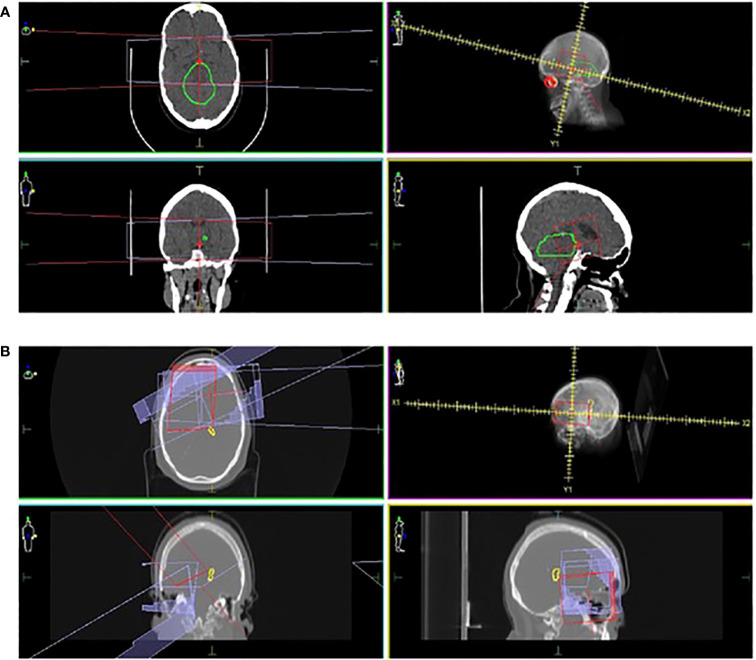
Locations of second brain malignancies relative to the treatment field. CT images in three planes, radiation treatment plans and the locations of second brain tumors are shown. In one patient (A), the second tumor (marked as green) is observed within the radiation field (marked in red and purple). In a second case (B), an anaplastic astrocytoma (marked as yellow) occurred on the edge of the radiation field (marked in red and purple).
